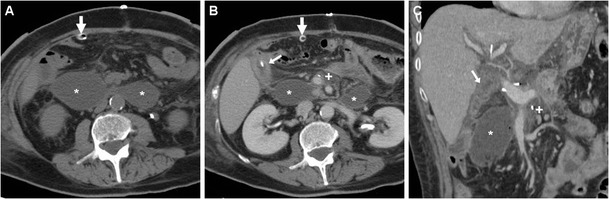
Non-infected postoperative collections in a 71-year-old man following PD for duodenal adenocarcinoma, complicated by intraoperative haemorrhagic shock. During prolonged hospitalisation, unenhanced (a) and postcontrast (b, c) CT images showed “saddlebag”-shaped retroperitoneal collection (*) with homogeneous fluid attenuation, thin walls. Note usual appearance of the MJL (*arrows*), scanty mesenterial fluid (+), drainage still in place (*thick arrows*). The patient ultimately recovered without additional procedures
